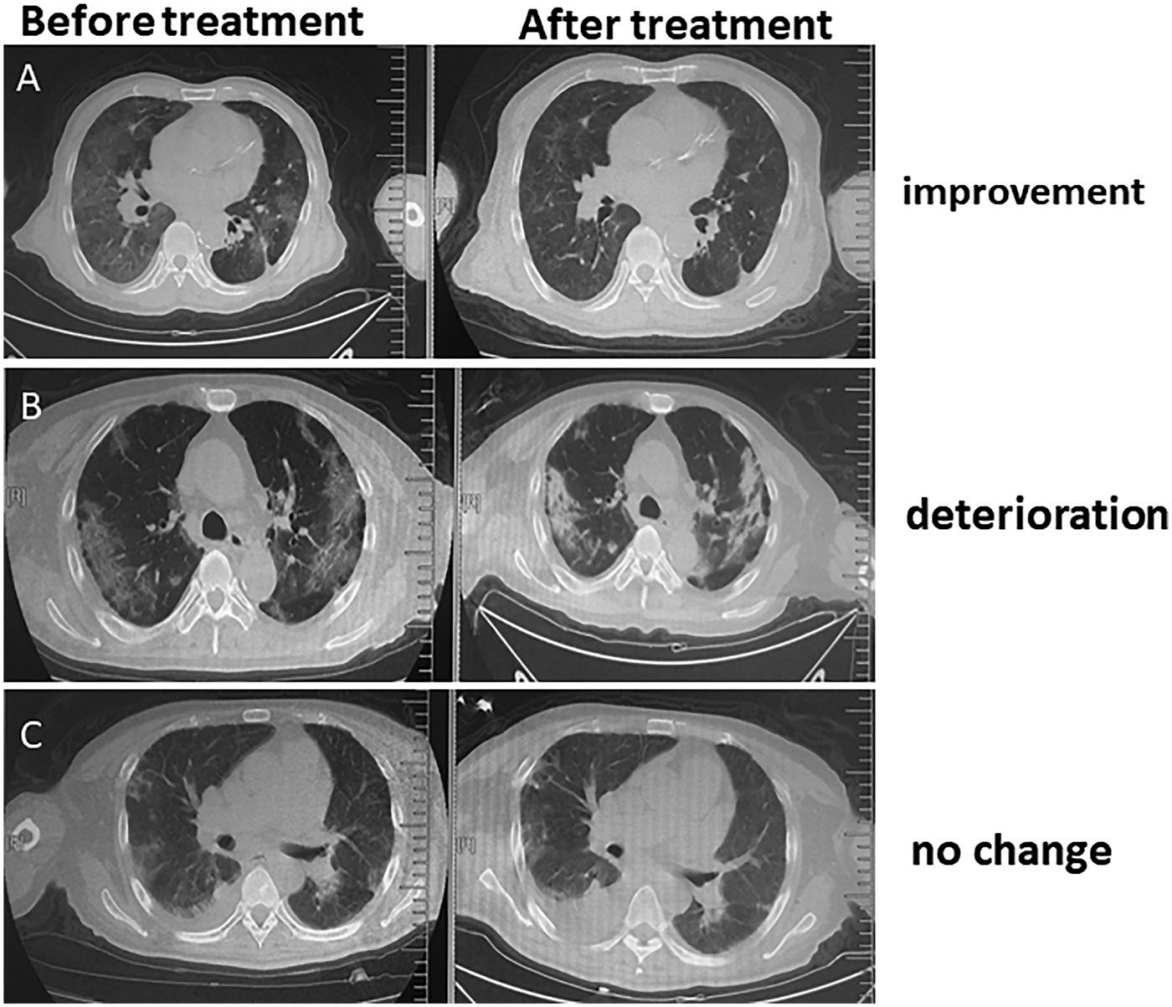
Typical CT scanning imaging features for HD patients infected with COVID-19 before and after treatment. (A) Improvement; (B) deterioration; (C) no change.
